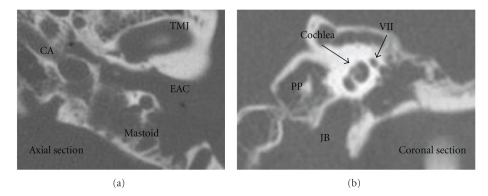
CT. The tympanum and mastoid were filled with an isodensity shadow with bone erosion. The wall of the carotid artery and jugular bulb appeared to be thick and erosive. CA: carotid artery, JB: jugular bulb, TMJ: temporomandibular joint, EAC: external auditory canal, VII: the seventh nerve, PP: Petrous pyramid.
